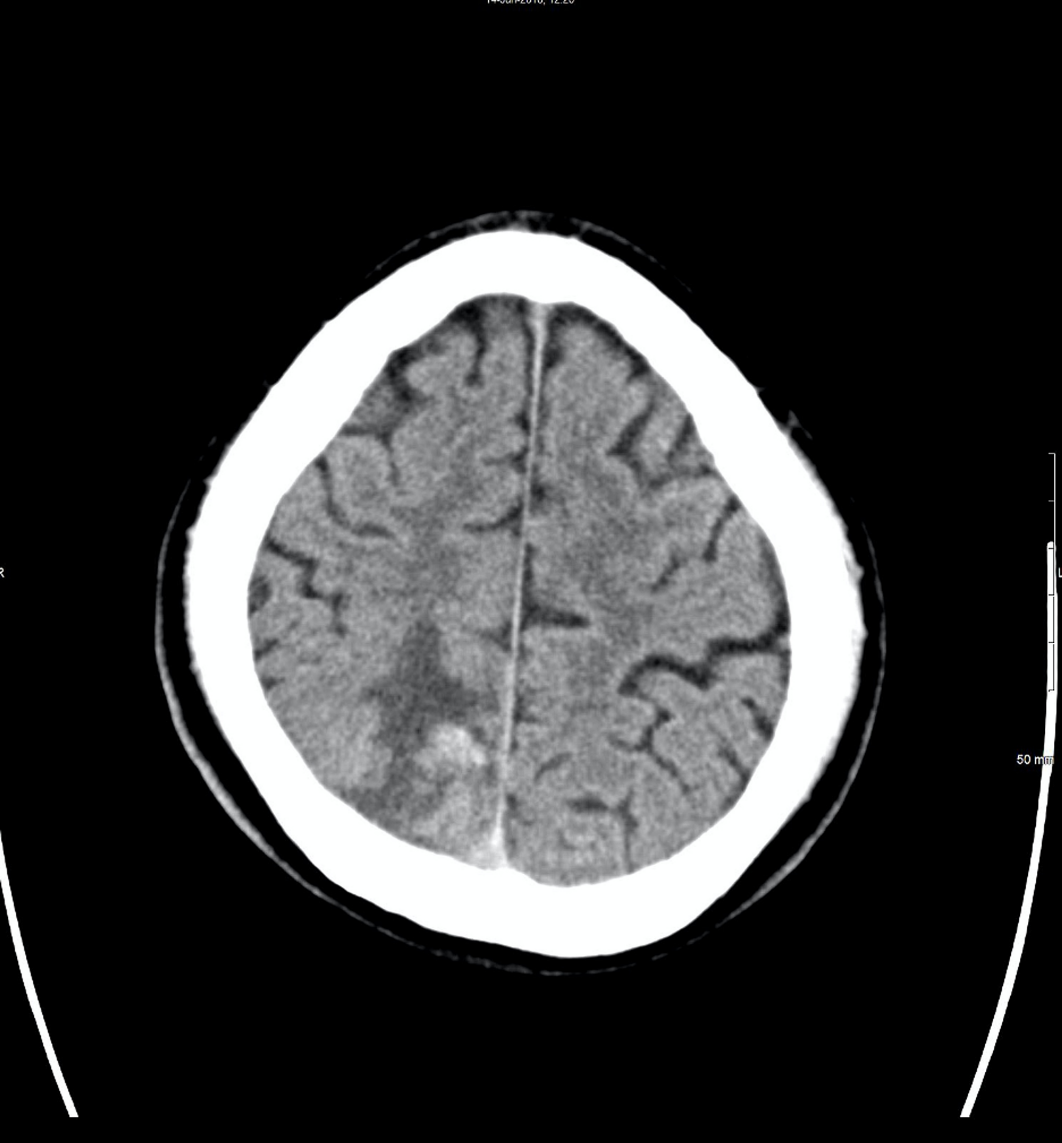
CT head, axial view. 1.0 cm hemorrhage at the gray-white junction of the posterior superior mesial right parietal lobe with surrounding edema.
